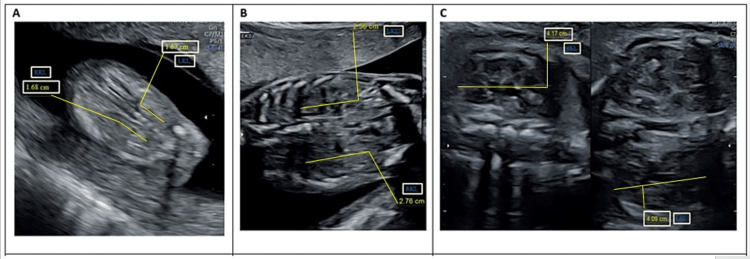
Ultrasound measurements of fetal kidney length at different gestational weeks: (A) 18th week, (B) 25th week, and (C) 37th week.
